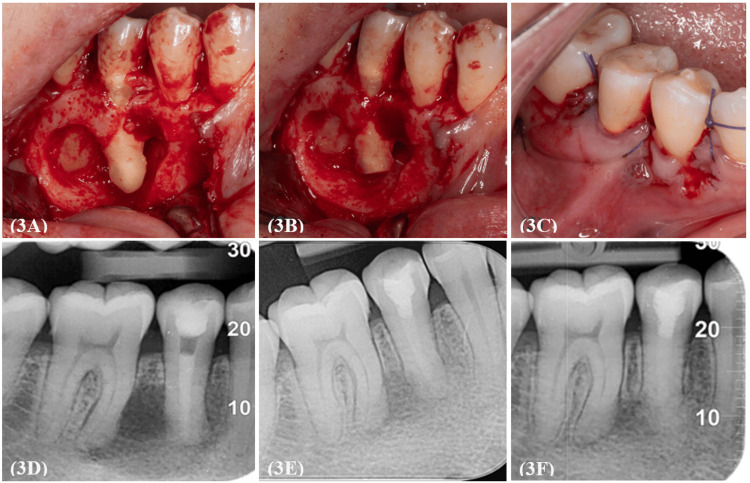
Peri-operative clinical photographs of periradicular surgery with post-operative and follow-up periapical radiographs (3A) Flap elevation revealed substantial resorption of the buccal cortical plate. (3B) Resection of 3 mm of the apical root to eliminate complex apical anatomical structures, including apical ramifications and lateral canals. (3C) Reapproximation of the flap with 4-0 Vicryl sutures. (3D) Immediate post-operative radiograph. (3E, 3F) Radiographic evidence of bone regeneration at the 6- and 12-month follow-up respectively, indicating periapical healing.
